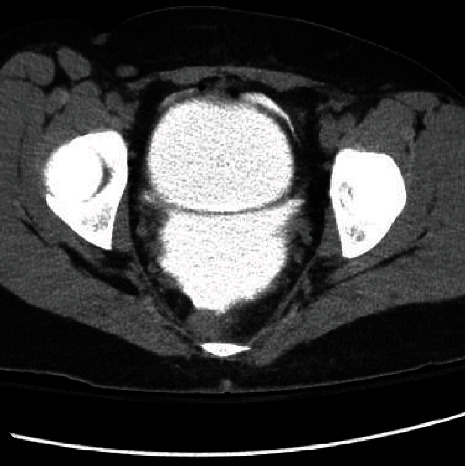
CT cystogram showing contrast extravasation into peritoneum and a small amount of free peritoneal air.
